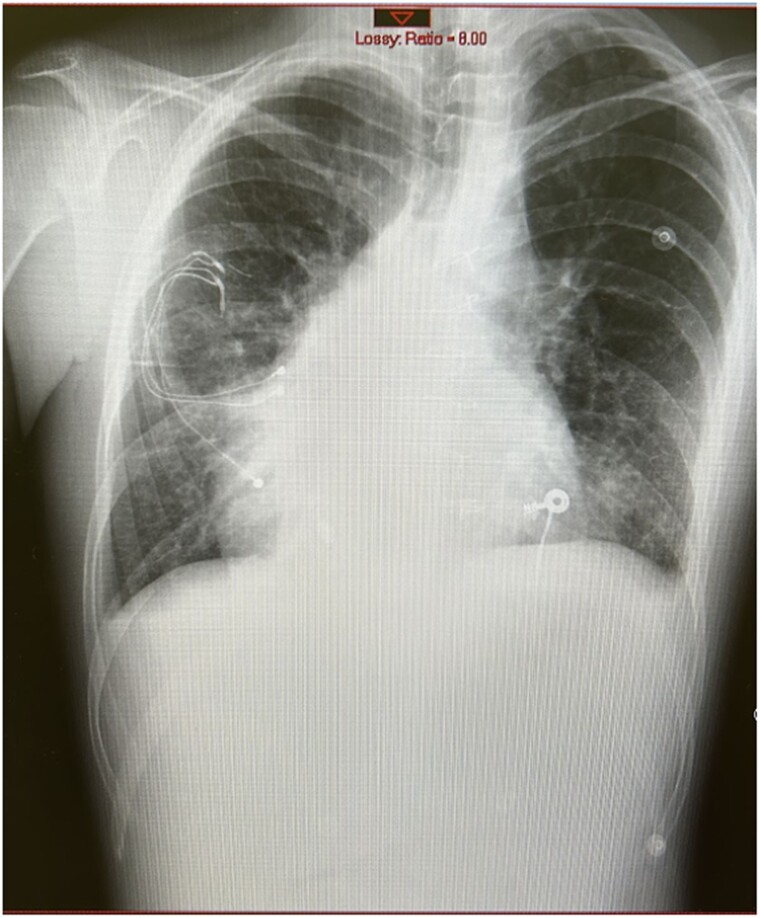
Anterior-to-posterior chest X-ray showing dextrocardia and abandoned epicardial leads of the cardiac resynchronization device.
